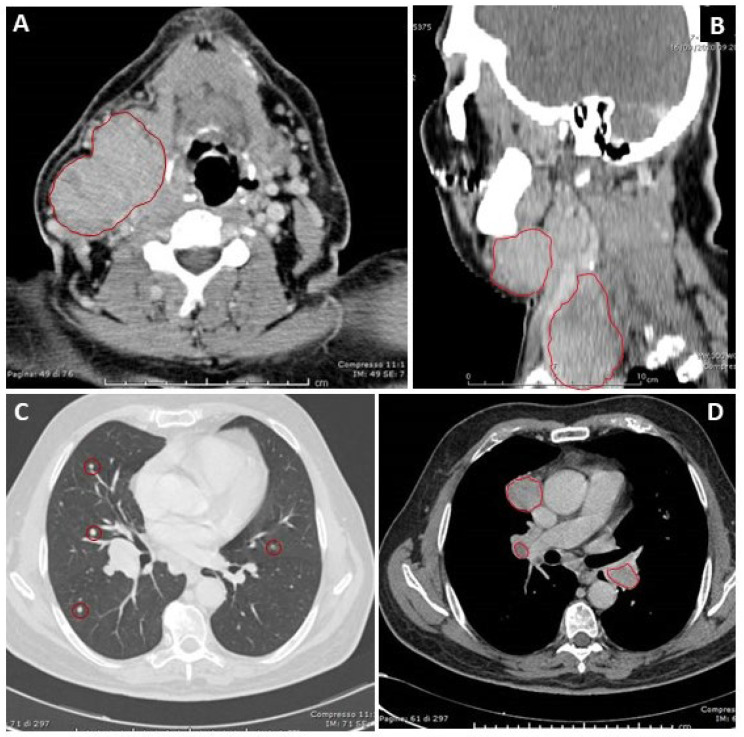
Representative images of contrast-enhanced total body CT: (A) Multiple confluent nodular lesions in right latero-cervical region, of overall size 56 × 58 mm, reaching superficial structures of neck and invading peri-thyroid muscles. (B) The nodular lesion in supraclavicular region (47 × 35 mm) invading muscles, causing compression and likely invasion of the internal jugular vein, and displacing anteromedially the right common carotid artery. (C) Multiple metastatic nodular lesions at midfields of lungs bilaterally. (D) Ilo-mediastinal lymphadenopathies (largest of 33 × 37 mm).
